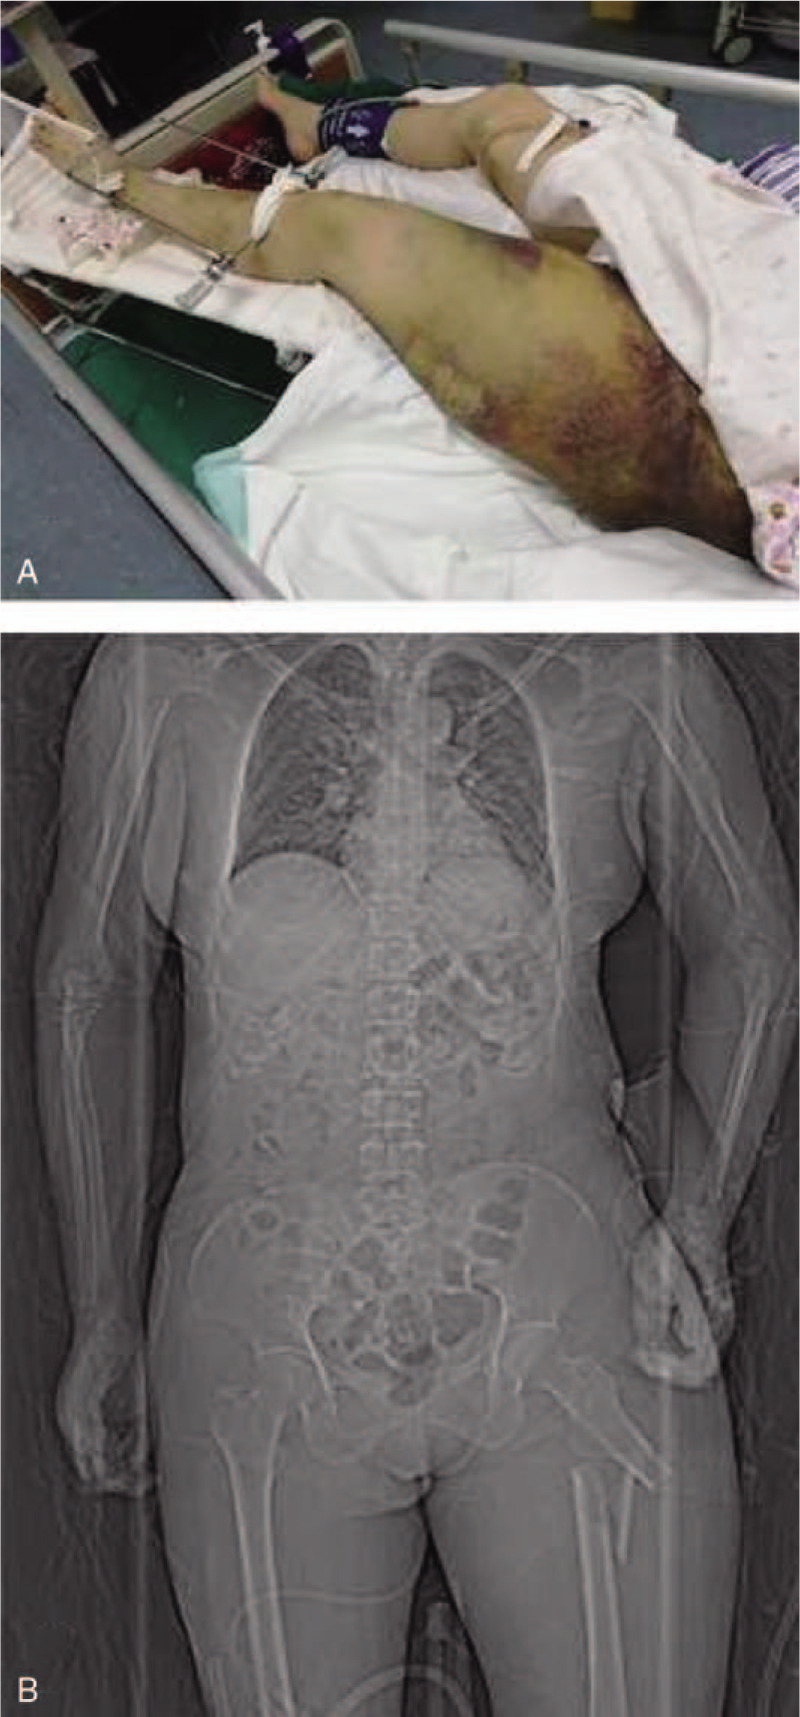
The left femur was fixed by external fixation (A); CT scan of the left femur (B) showing a comminuted fracture.
